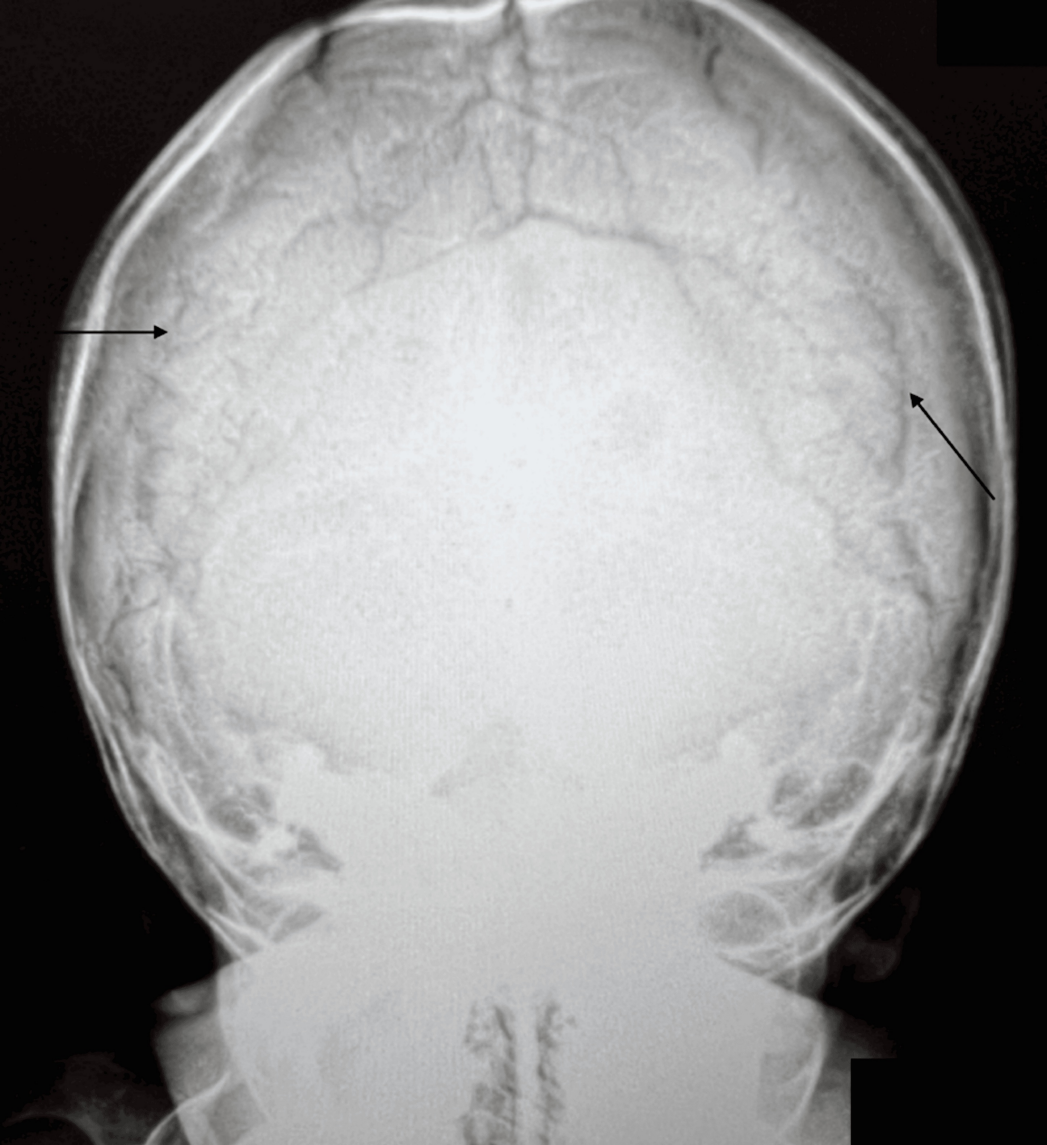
Skull X-ray. Arrows indicate multiple lambdoid Wormian bones.
